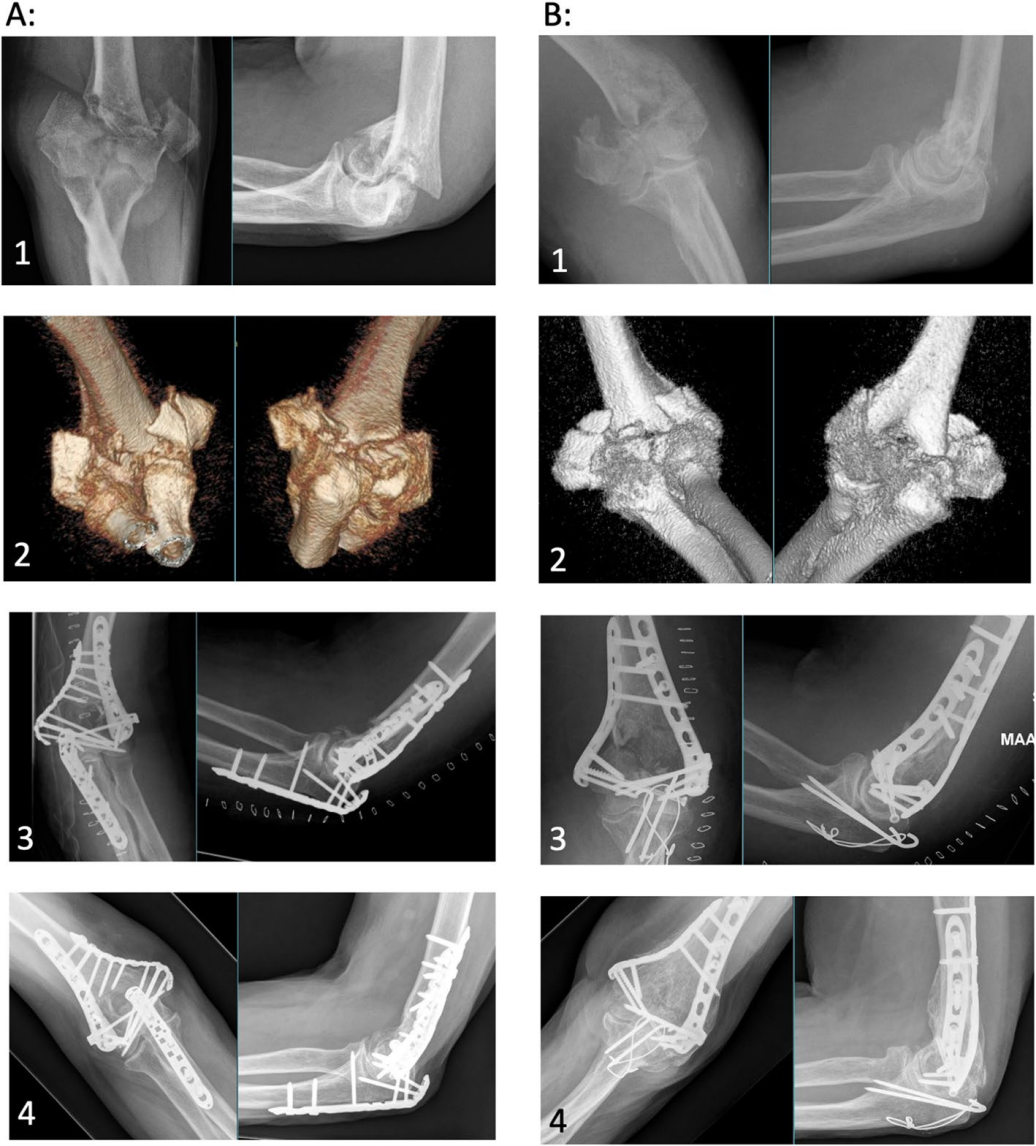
Radiographs of patients with typical fractures resulting in. A a good result (OES 94, flexion–extension arc 125 degrees, MEPS 100, qDASH 10) and B stiff elbow with moderate outcome (OES63, flexion–extension arc 75 degeres, MEPS 60, aDASH 38). 1: postinjury radiograph. 2: postinjury CT 3D reconstruction. 3: postoperative radiograph. 4: radiograph at follow-up (A: 1,8 years, B: 2,5 years)
